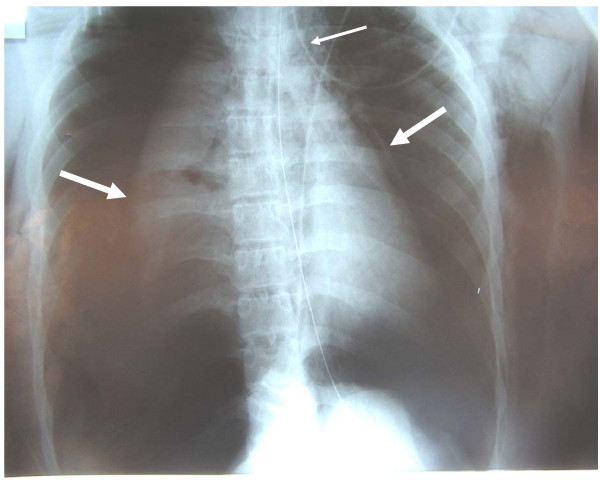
Bedside chest radiography performed immediately after admission. Bilateral pneumothorax (large arrows), pneumomediastinum (thin arrows) and extensive subcutaneous emphysema are visible.
